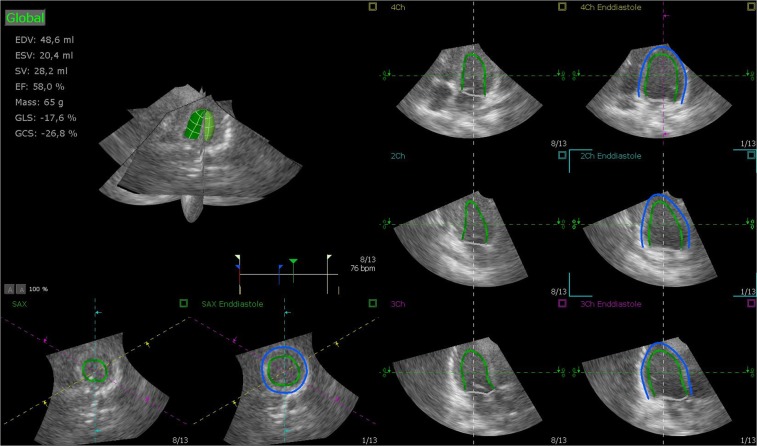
Sample images of the heart by RT3DE (software Tomtec). Two-dimensional slices from a representative real-time 3D echocardiographic data set with endocardial (green) and epicardial (blue) borders of the left ventricular myocardium.
